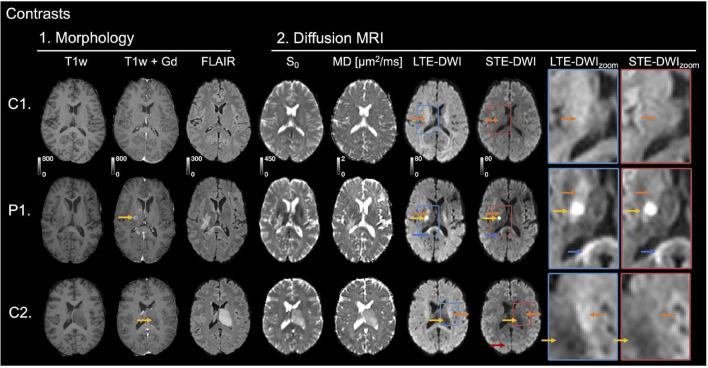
Investigated image contrasts. Three contrasts were related to morphology: T1w, T1w contrast-enhanced by Gadolinium (T1w+Gd), and T2w FLAIR. Four were related to DWI: *S*_0_, mean diffusivity (MD) and high *b*-value diffusion-weighted imaging (DWI) (*b*
